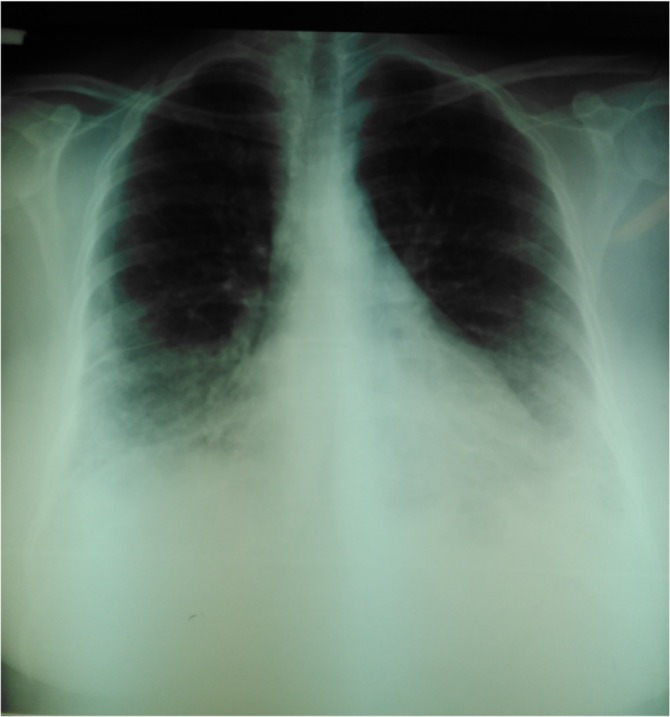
Chest x-rays showing increased markings in lower lung fields.
